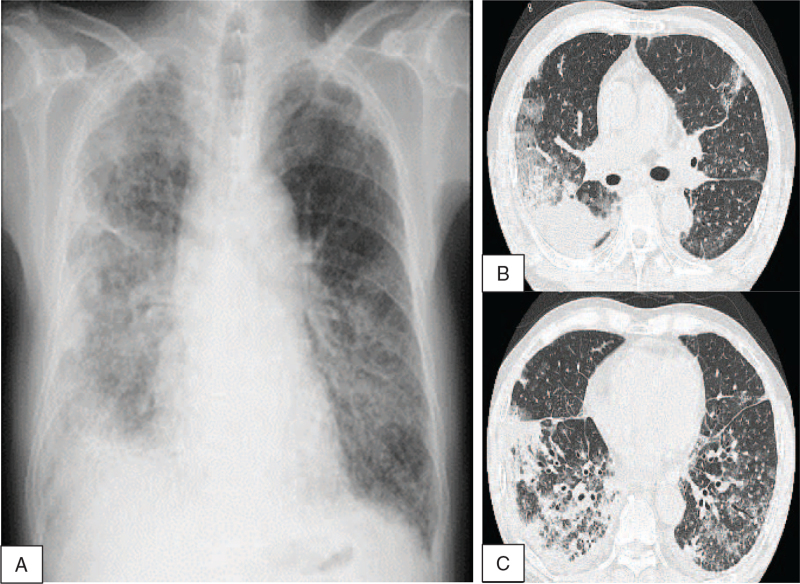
(A) Chest radiography shows consolidation of the bilateral lung fields. The right costophrenic angle is dull. (B and C) Computed tomography of the chest reveals bilateral centrilobular micronodular opacities compatible with silicosis, and it also shows enlarged ground-grass shadows, infiltrative shadows and increased pleural effusion, compatible with acute interstitial pneumonia (lupus pneumonitis) and pleuritis.
